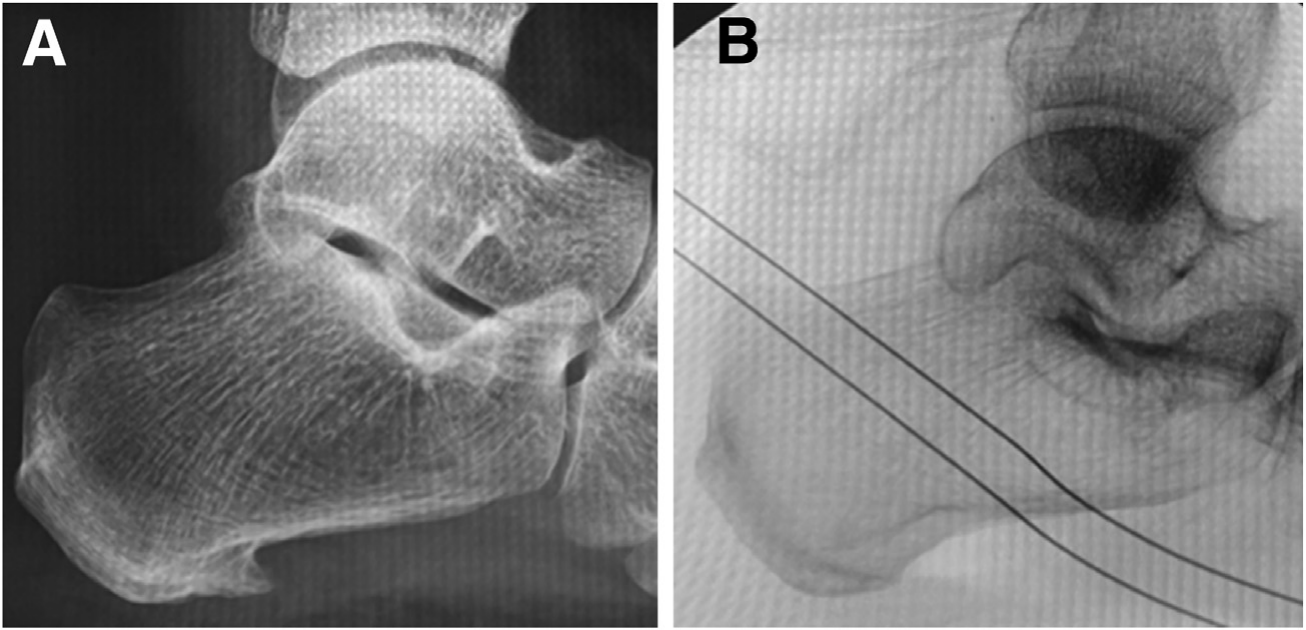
(A) Preoperative radiograph showing calcaneal spur. (B) Intraoperative image confirming complete resection of calcaneal spur. The patient is positioned supine (left foot).
